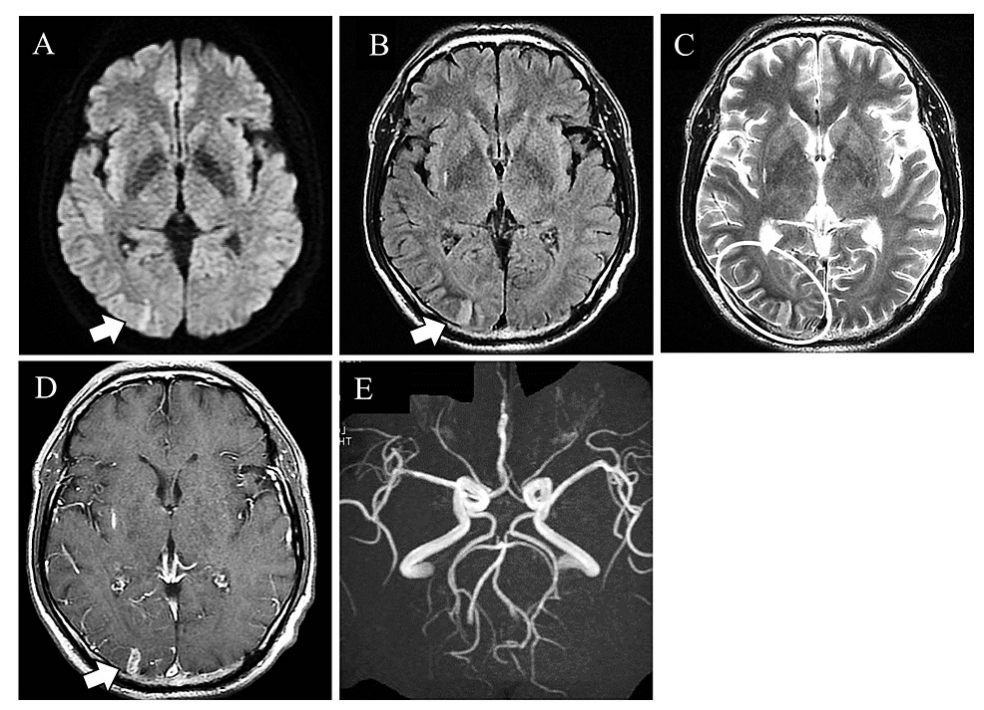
Contrast-enhanced magnetic resonance imaging (MRI). The occipital lobe showed cortical high intensity on diffusion-weighted image (A), fluid-attenuated inversion recovery (B), and a subcortical low-signal on T2 (C). The occipital lesion showed gadolinium enhancement (D). MR angiography showed no vessel stenosis or occlusion (E).
